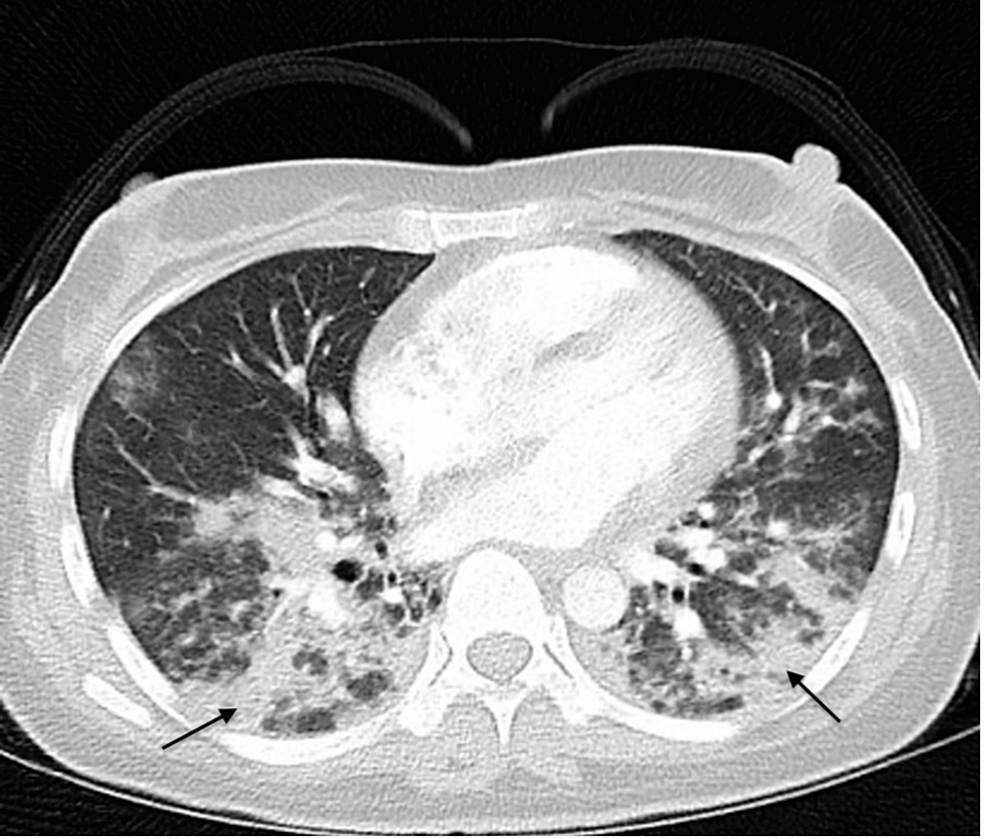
The CT of the chest was significant for extensive ground glass/alveolar opacities (see arrows).
